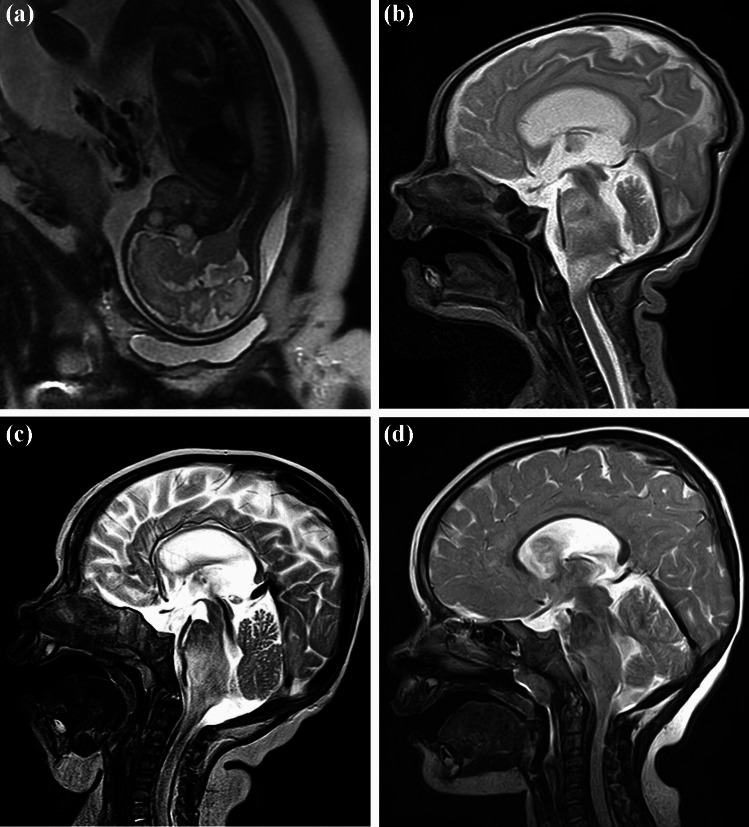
Case 4. Follow-up. All T2-weighted images, sagittal plane; intrauterine MRI obtained 24 days before birth (a), at the 12th day of life (b), at the age of 5 months (c), and 2 years old (d). After detecting the tumor with ultrasound, the brainstem glioma is already visible prenatally on MRI, demonstrating an increased volume at day 12 of life and a spontaneous and continues regression documented until the second year of life
